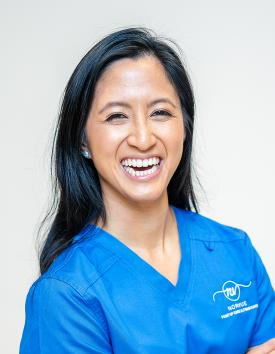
Dr Ai Phi Thuy Ho is a cardiology fellow at Ostfold County Hospital Kalnes, specializing in cardiovascular care, and has a great passion for point-of-care ultrasound (POCUS). Educated in Norway (MD, 2015), she is the founder and CEO of NorVue, a platform providing POCUS training for healthcare professionals. Dr Ai Phi Thuy Ho is passionate about advancing diagnostic accuracy and clinical education. She is also actively involved in humanitarian work across Africa and contributes to a US-based non-profit focused on global health outreach and medical teaching. Her dedication to innovation, learning, and service reflects a deep commitment to improving patient care both locally and globally.
